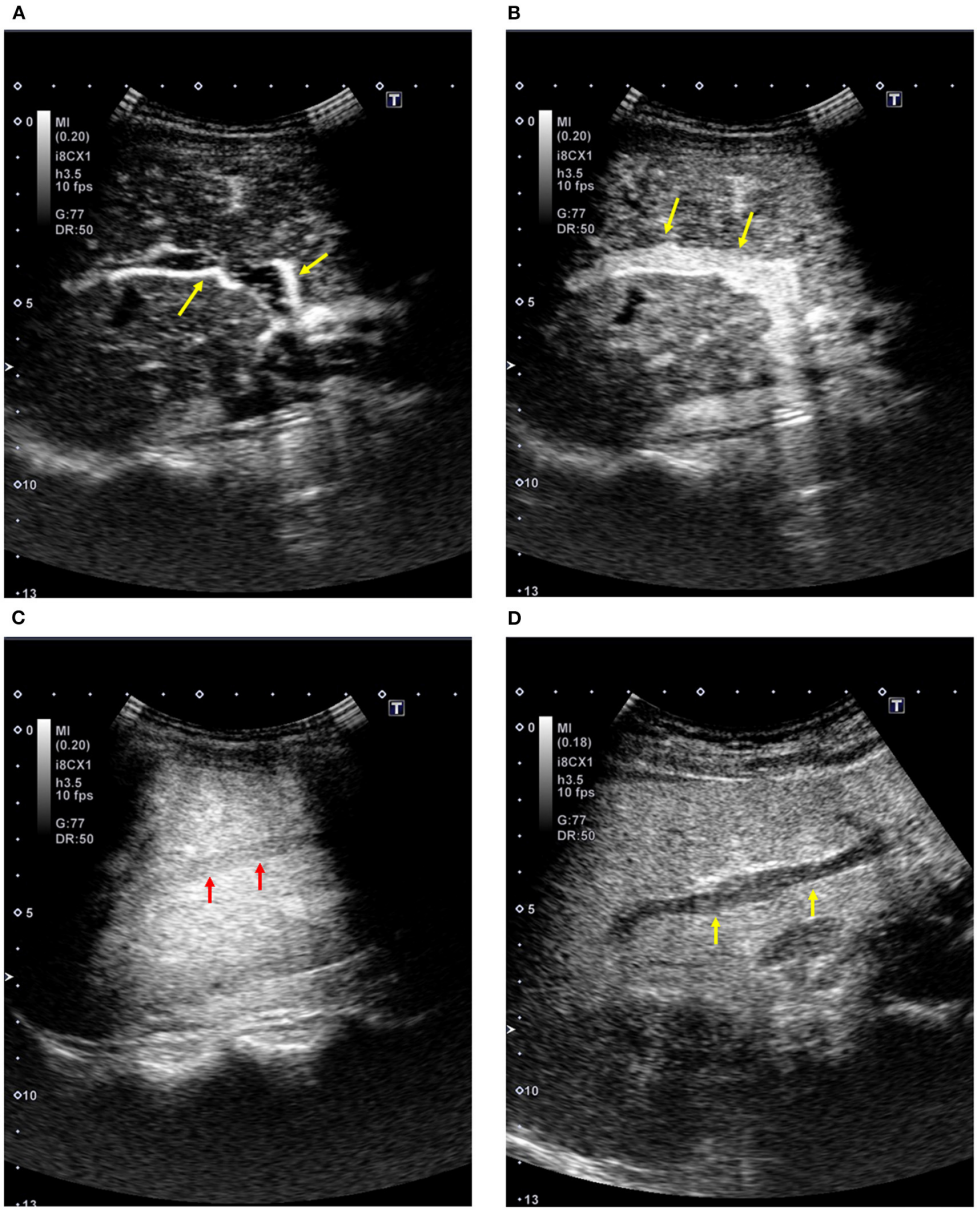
Contrast-enhanced ultrasound of the liver (Sonazoid; healthy subject, 51-year-old women). (A) Arterial phase: The sonogram shows an enhancement in the right hepatic artery (arrows). (B) Portal venous phase: The sonogram shows an enhancement in the right portal vein (arrows). (C) Contrast enhancement in the hepatic vein: The sonogram shows an enhancement in the right hepatic vein (arrows). (D) Post-vascular phase (12 min after the injection): The sonogram shows homogeneous enhancement in the liver parenchyma with the absence of enhancement in the intrahepatic vessels (arrows).
